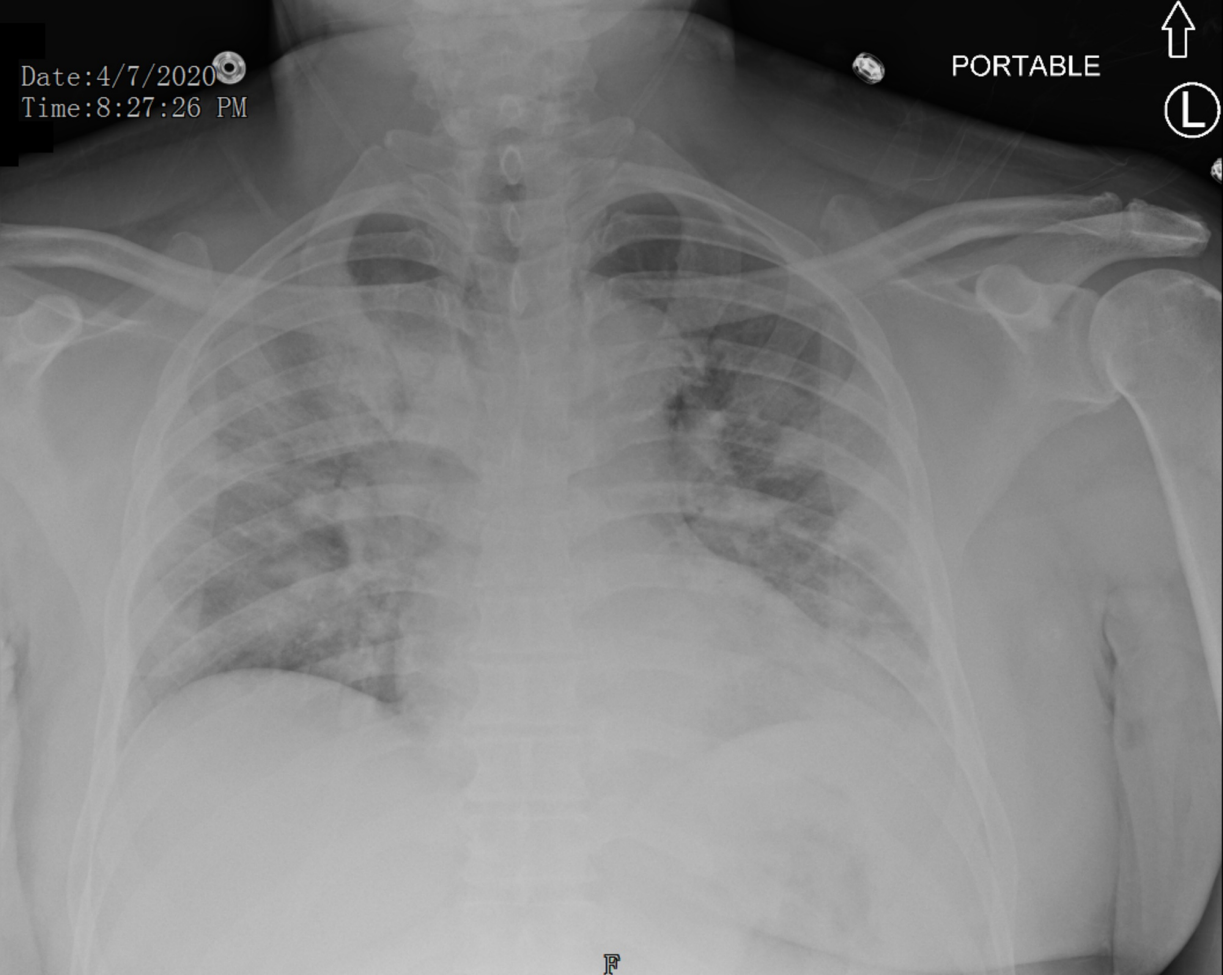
Chest X-ray (day 2) revealed bilateral infiltrates
